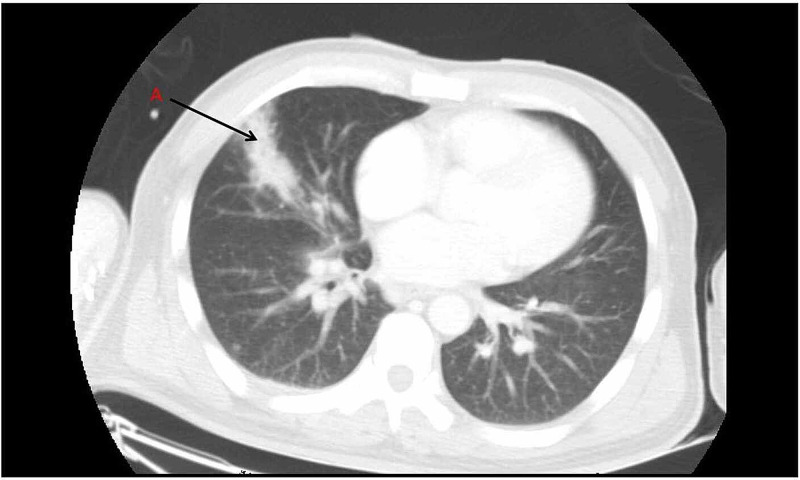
CT chest showing right lung opacity A: Right middle lobe opacity
